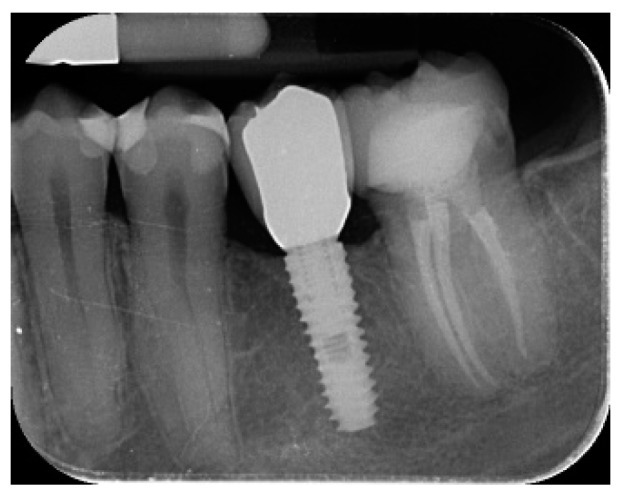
18 months radiographic follow-up.
